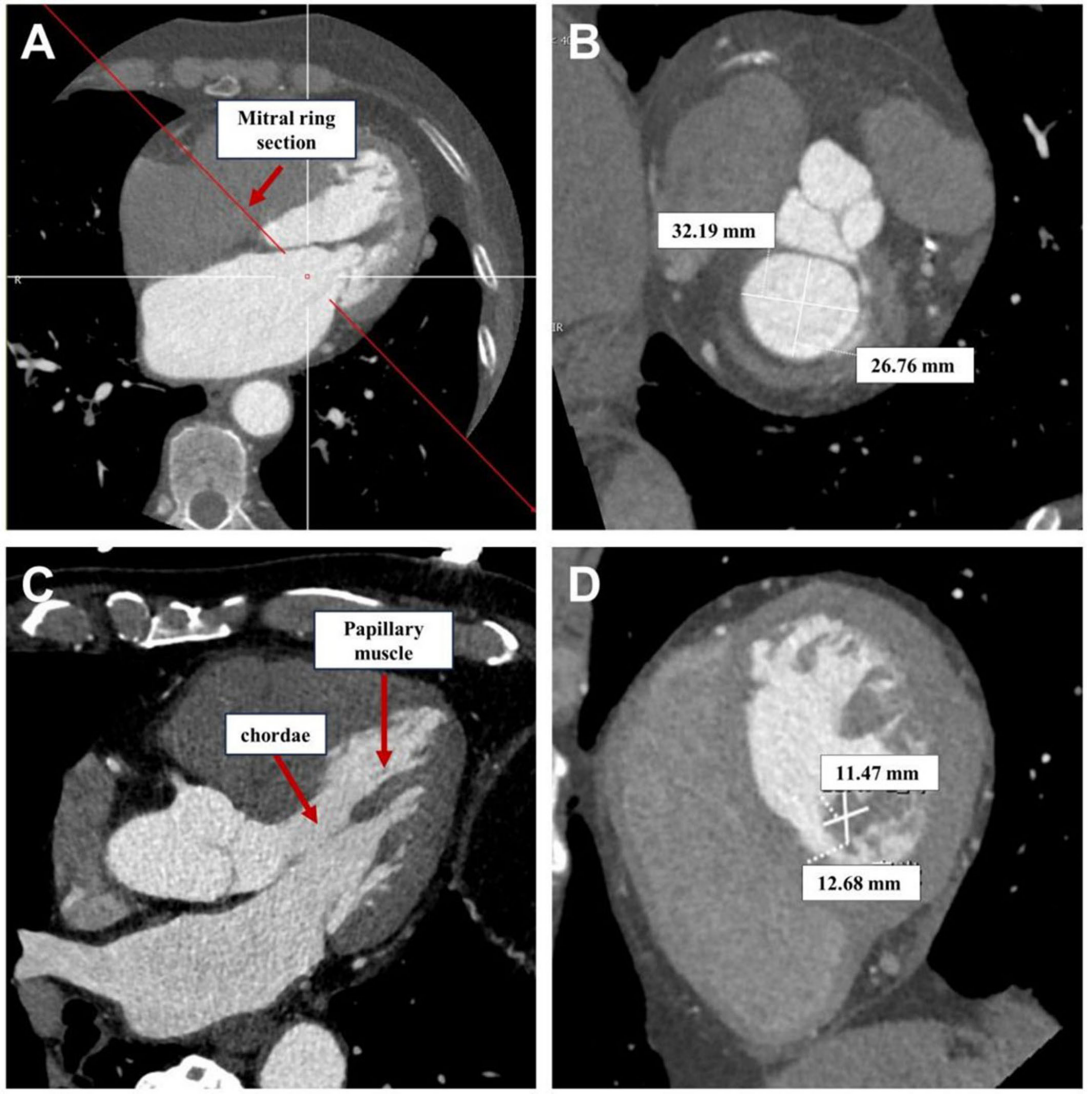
Multiplanar reformation after cardiac CT scan for visualizing
